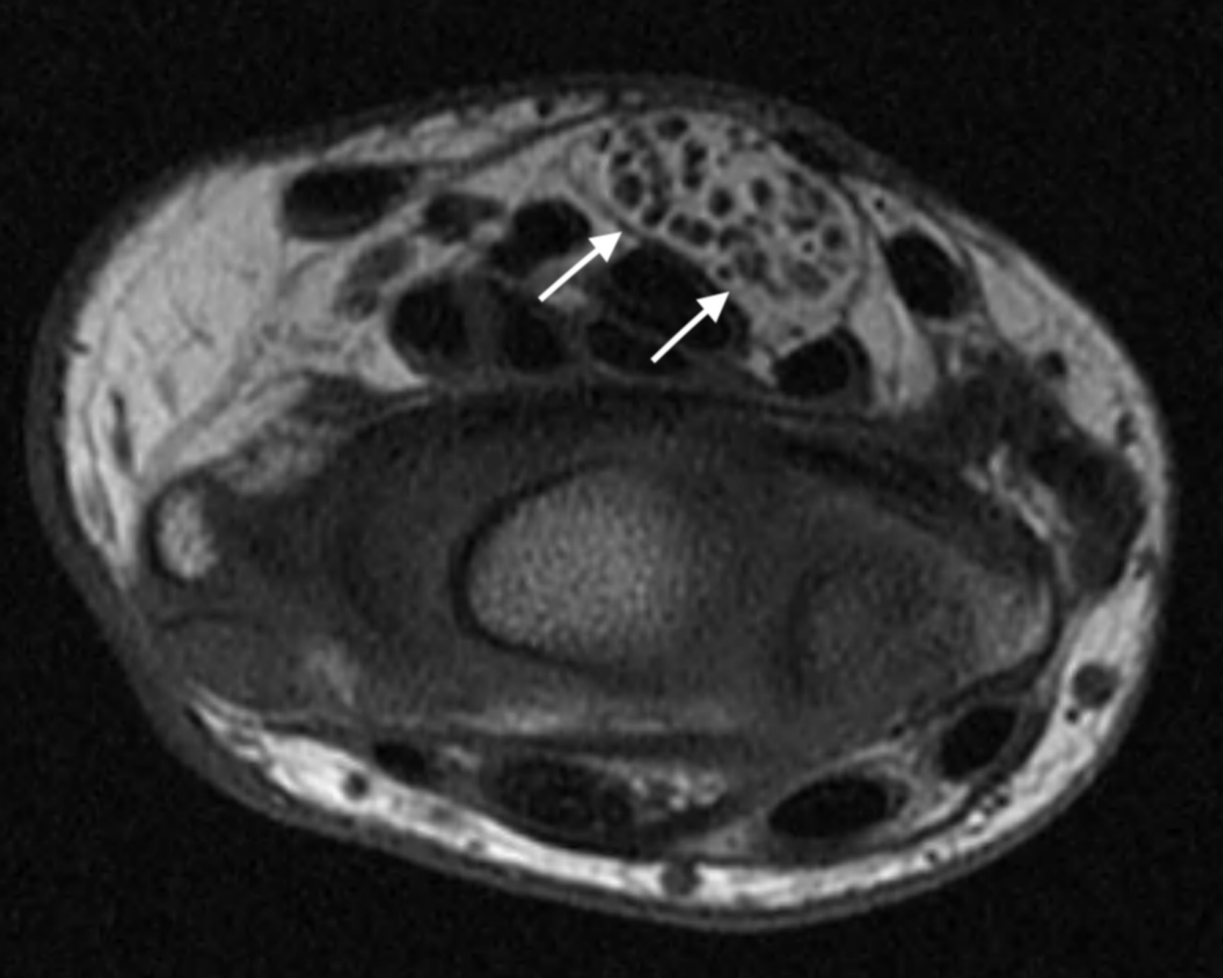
T1 magnetic resonance imaging (MRI) of the left median nerve at the wrist White arrows median nerve
